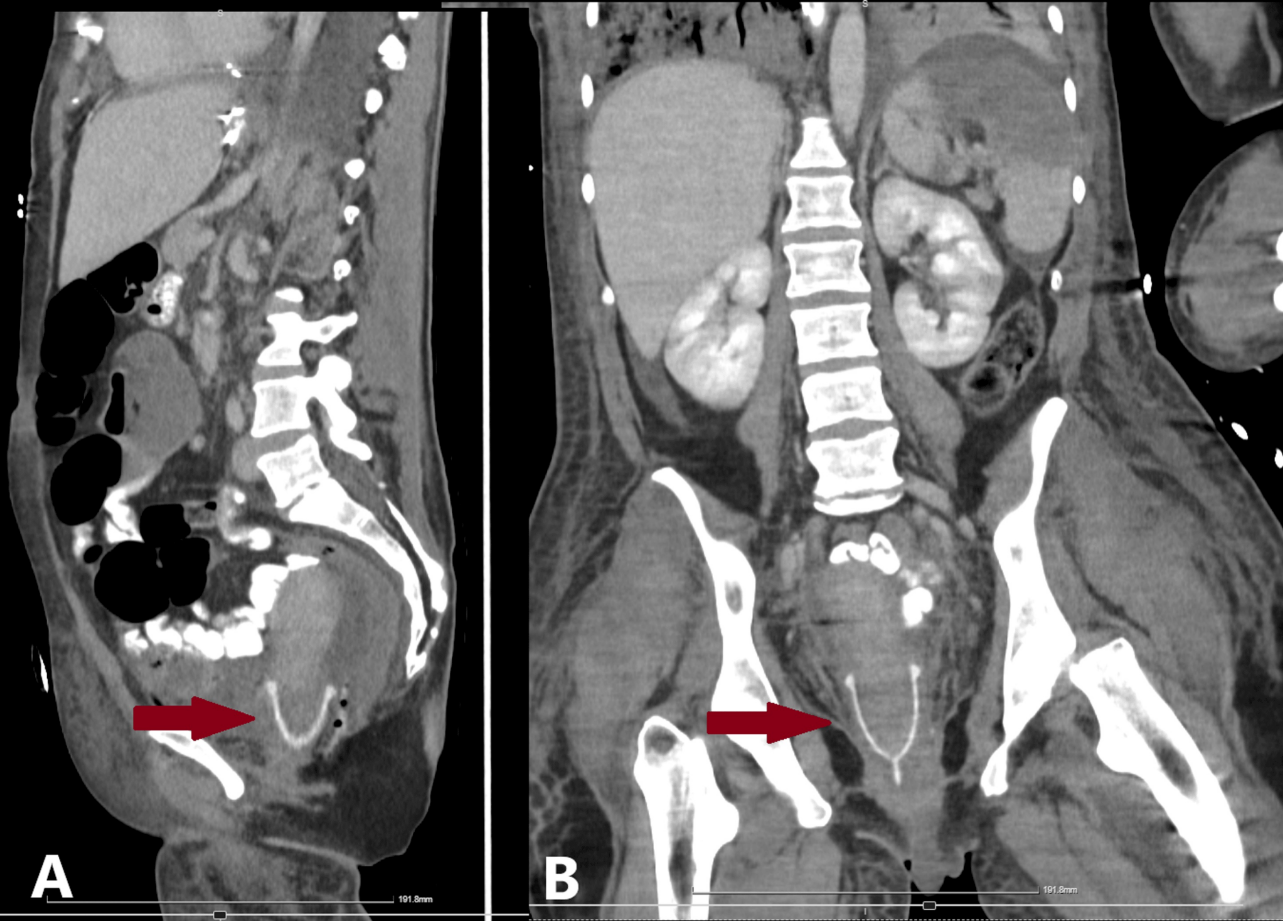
CT of the abdomen and pelvis. (A) Sagittal view; (B) Coronal view Visible menstrual cup located at the cervix (red arrows). CT, computed tomography
